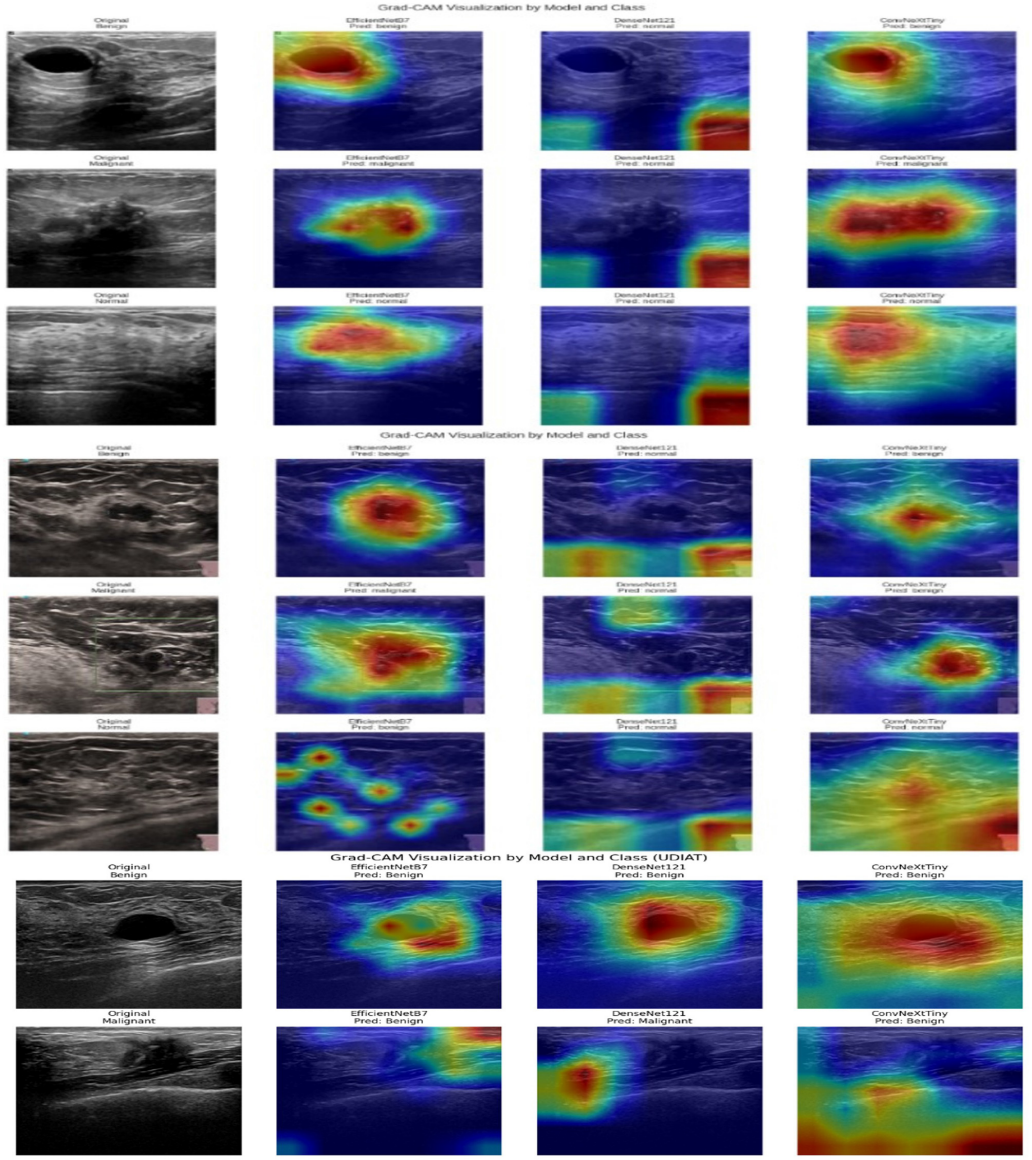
Grad-CAM visualization on benign, malignant, and normal images on (Top three) BUSI, (Middle three) BUS-UCLM, and (Bottom two) UDIAT datasets. Column 1 presents the actual ultrasound images; subsequent columns exhibit the equivalent heatmaps produced by EfficientNetB7, DenseNet121, and ConvNeXtTiny, respectively. Warmer colors like red and yellow signify areas of significant model attention that most substantially influenced the categorization decision, while cooler colors like blue denote minimal contribution.
